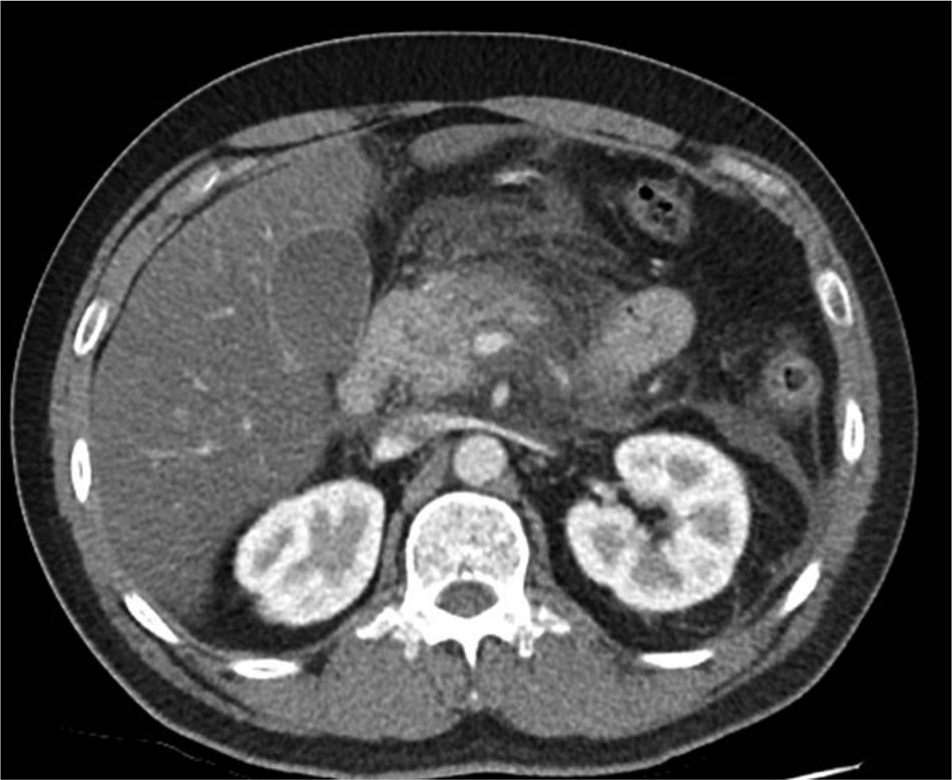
Axial CT image demonstrating body and tail pancreatitis with fat stranding.
